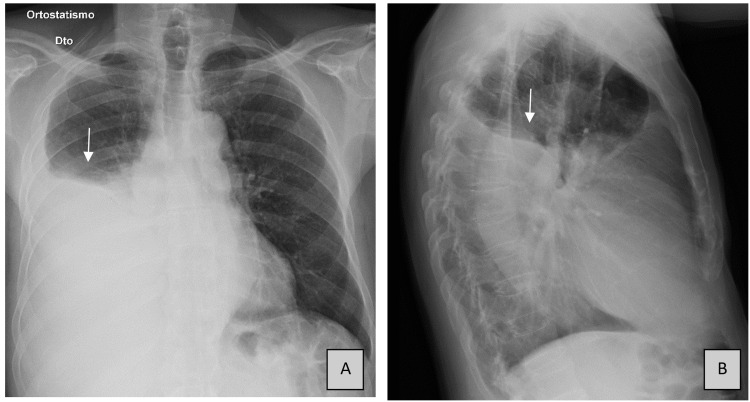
Chest radiographs. Posteroanterior (panel A) and lateral (panel B) chest radiographs presenting a large right pleural effusion (arrows). Dto: Right.
